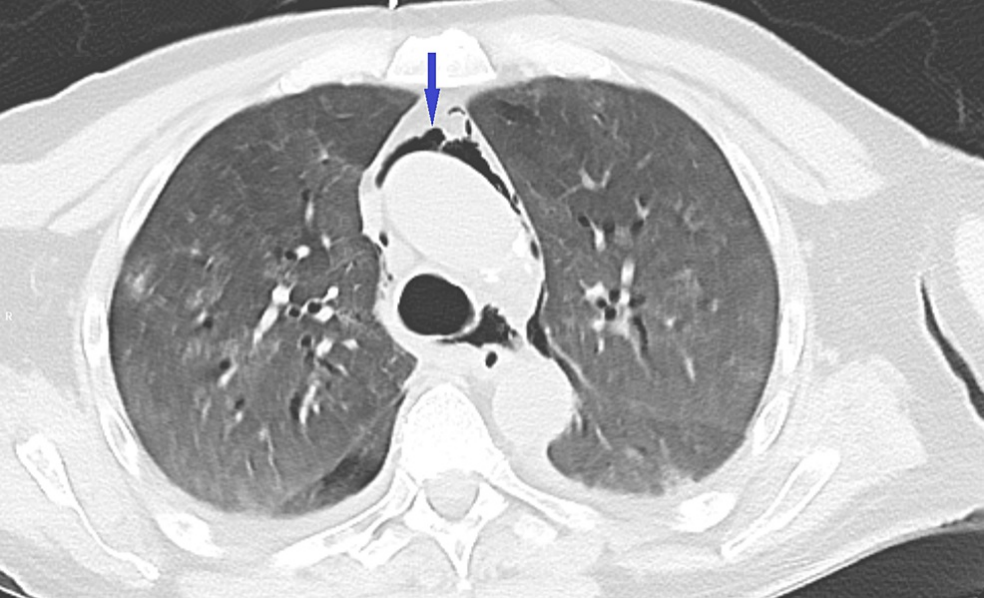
CT of the chest (axial view) showing pneumomediastinum.
